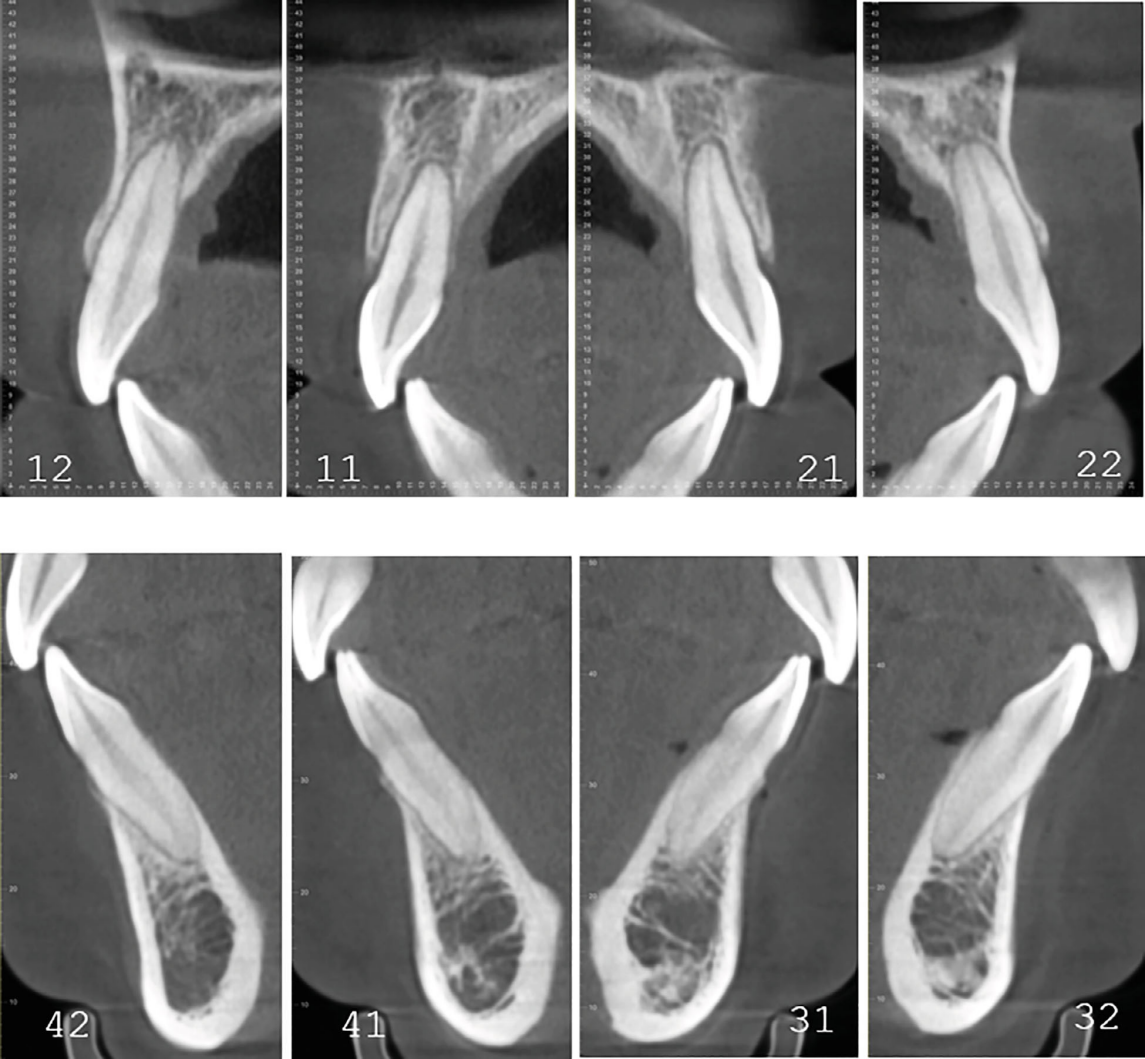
Posttreatment alveolar bone in labiolingual dimension in CBCT.
